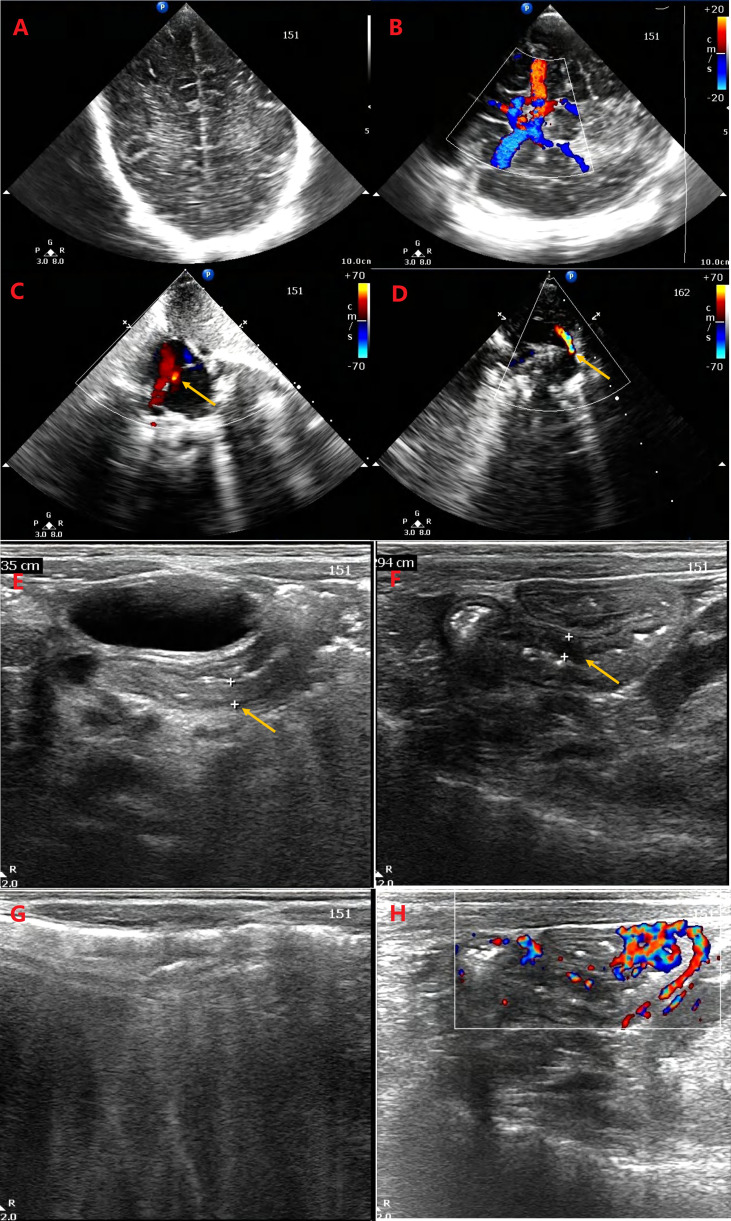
Ultrasonic Doppler resolution is applied for detecting the abdomen, heart, and brain. No obvious abnormality was detected on the brain sonogram (A, B). The patent ductus arteriosus (D) and patent foramen ovale (C) were found. There are partial bowel flatulence of epigastrium, partial intestinal thickening of colon (0.335 cm, 0.294 cm) (E, F), and a little of seroperitoneum. Intestinal gassiness (G) and blood flow in thickened intestinal wall (H) were detected.
